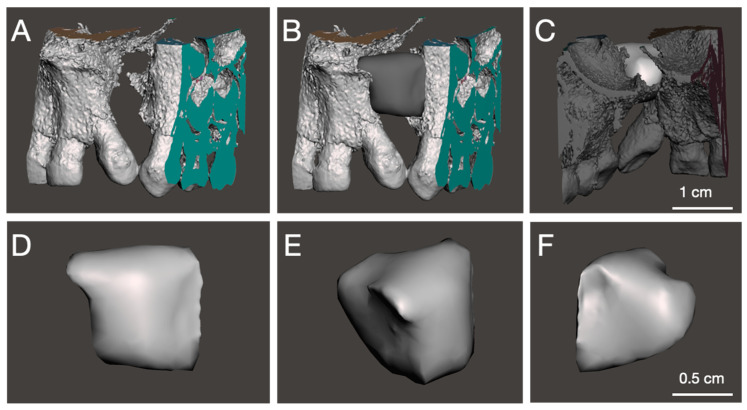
Example of design of a part from the CBCT seen in the previous figure. Initial situation (A) then with the part filling the critical defect in front (B) and posterior (C) view. Front (D), anterior (E), and posterior (F) views of the part.
